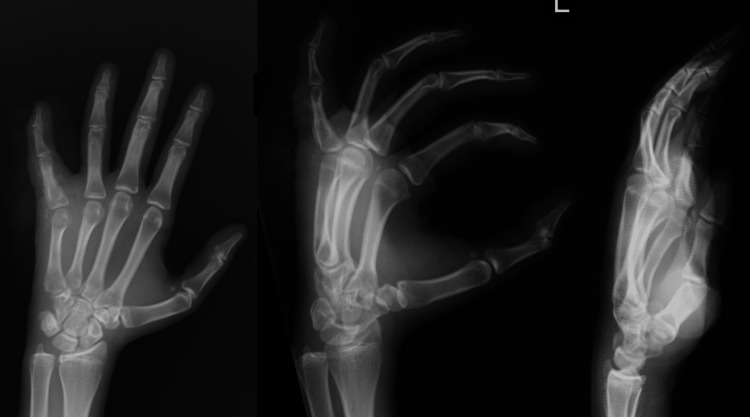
Post-operative anteroposterior, oblique, and lateral view radiographs of the left hand with resolution of the periosteal reaction of the proximal phalanx of the little finger
